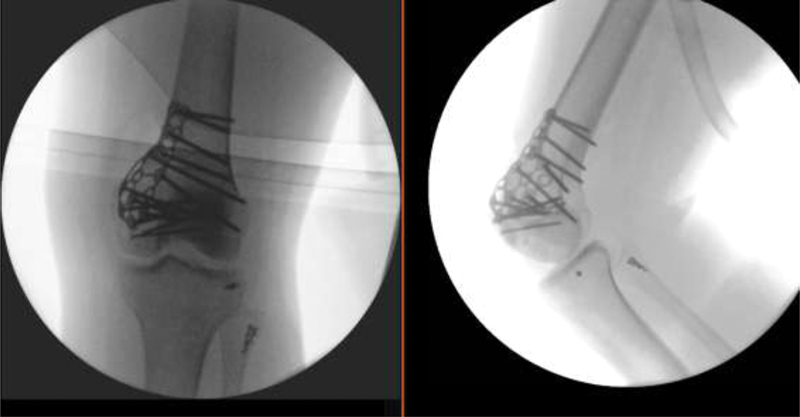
Anteroposterior and lateral view intraoperative radiographs.
